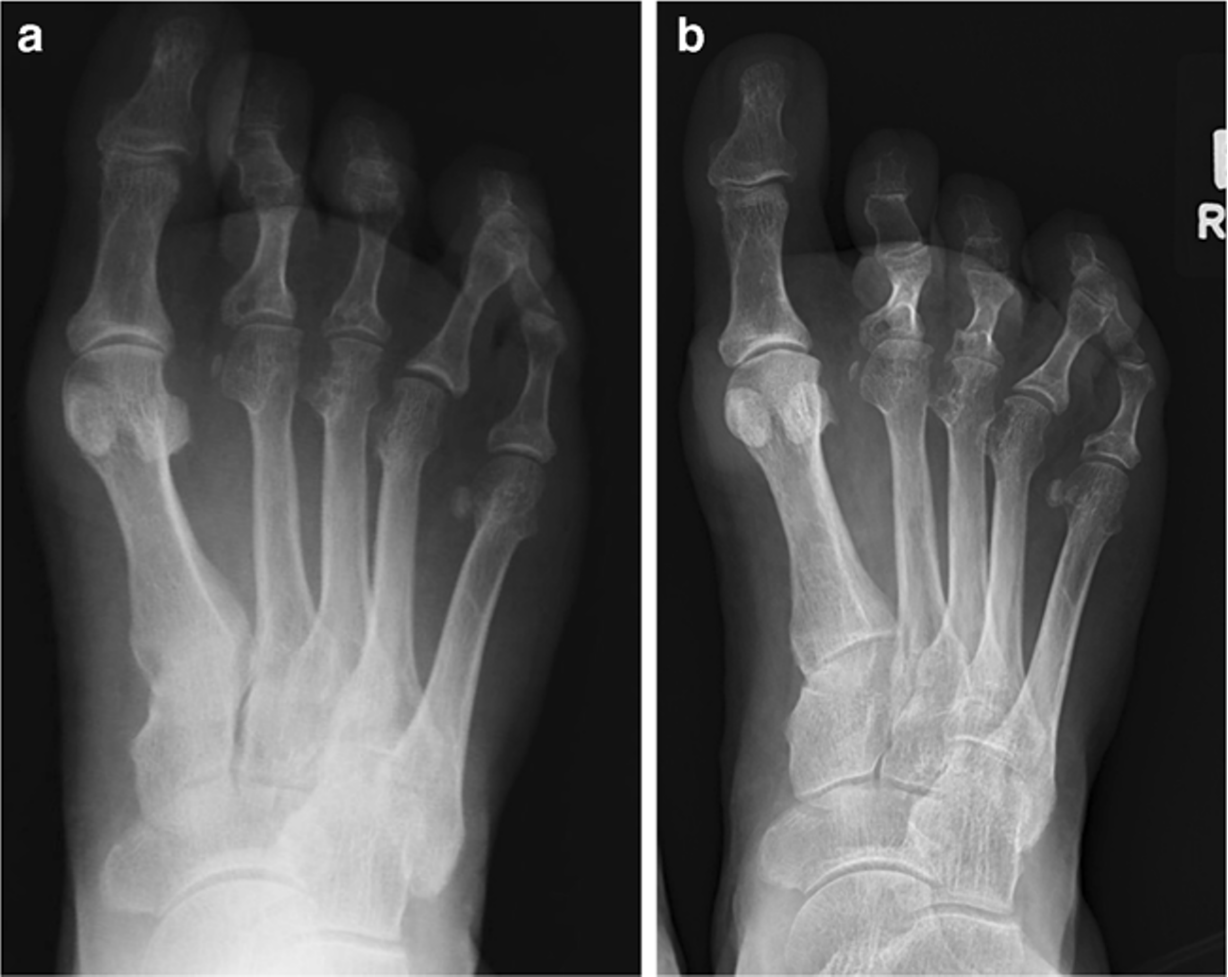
The non-weightbearing (a) and weightbearing (b) x-rays of the same patient. A 16° decrease in HVA and 3.4° decrease in IMA were observed
